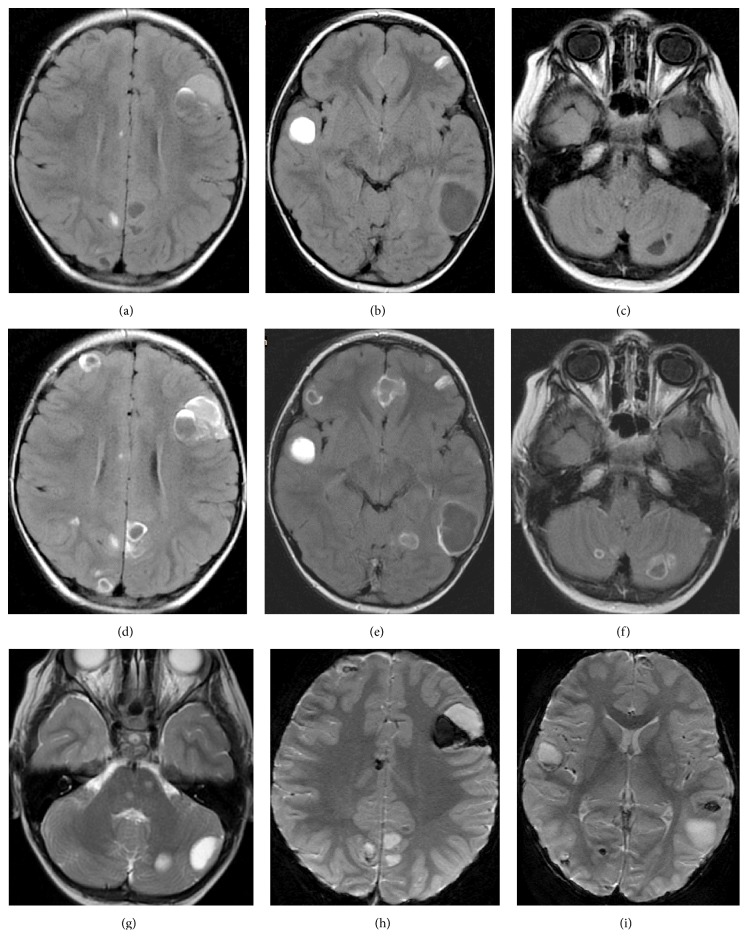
A 5 year-old-boy with foot rhabdomyosarcoma. Enormous number and heterogeneity of metastases form: cystic, hemorrhagic, solid, and leptomeningeal lesions disseminated in both cerebral and cerebellar hemispheres as well in the brain stem. MRI; FLAIR before (a, b, c) and after gadolinium administration (d, e, f); FSE/T2WI (g); GRE/T2*∗*WI (h, i).
